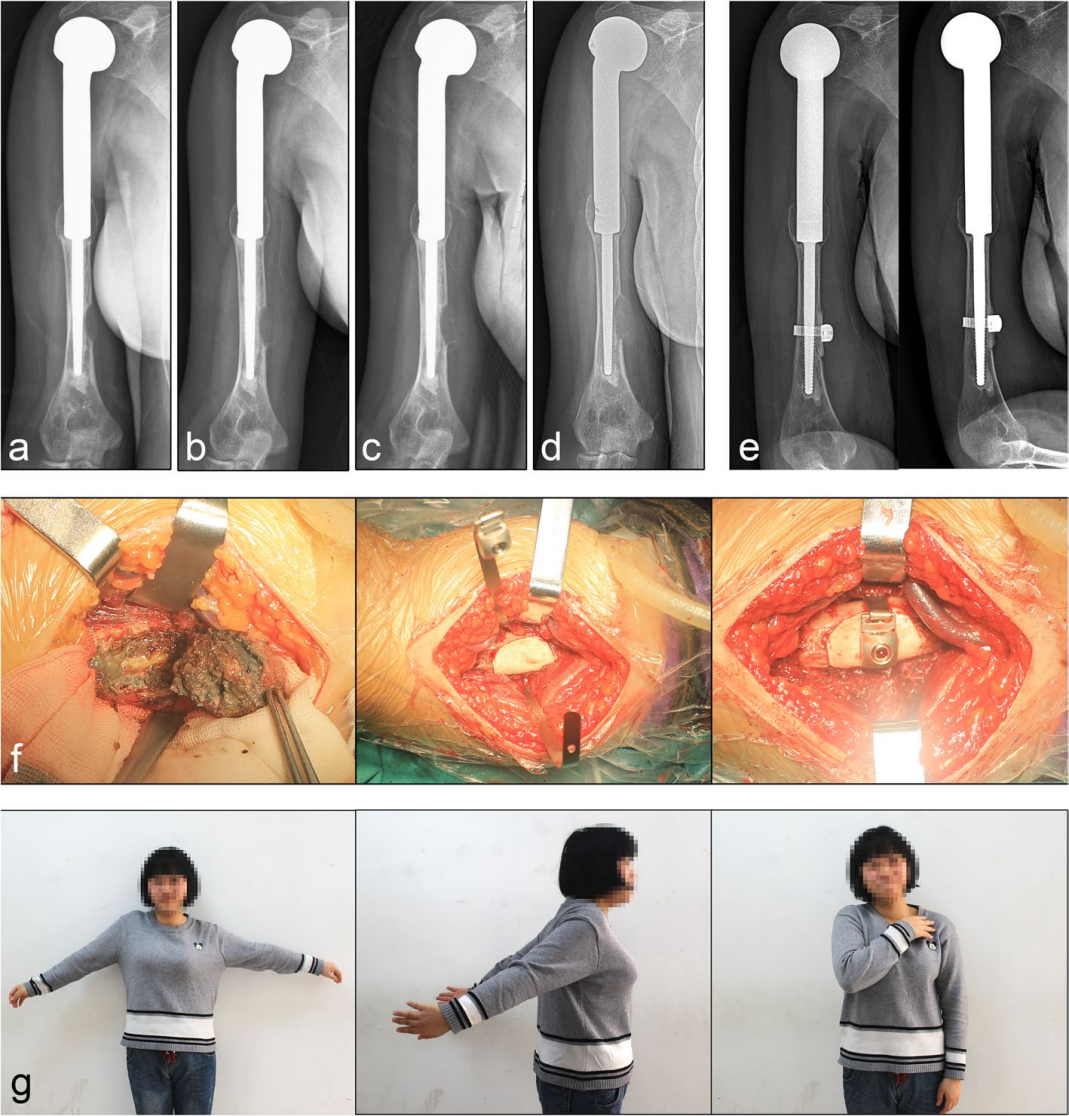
Giant cell granuloma of distal prosthesis was observed during follow-up. a–d Four consecutive X-ray reexaminations revealed osteolytic lesions of the distal humerus with slight enlargement. e Postoperative X-rays of lesion resection and fixation of allograft bone block with bone cerclage. f The lesion was excised, then an allograft of appropriate size was cut to repair the bone defect, and finally, the allograft was fixed with a bone cerclage. g Postoperative shoulder joint motion was satisfactory, especially shoulder abduction function
